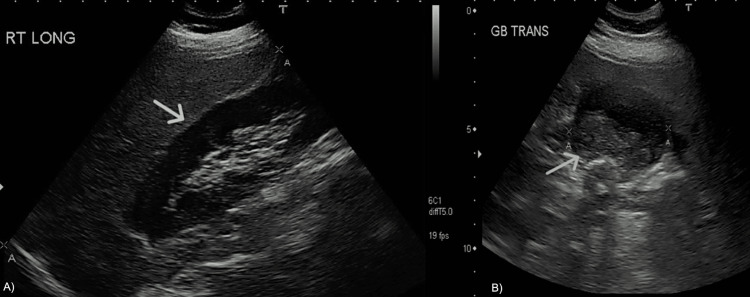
Right upper quadrant ultrasound demonstrating cholelithiasis with gallbladder distention (white arrow, Panel A) in the longitudinal plane and biliary sludge (yellow arrow, Panel B) in the transverse plane
